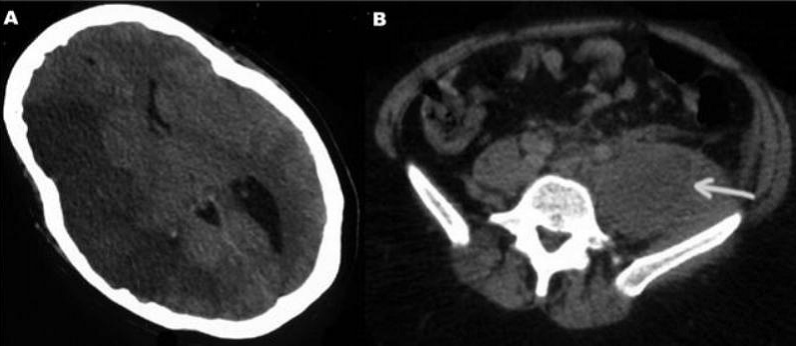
A) Brain computed tomography scan depicting a large right-sided ischemic region and cerebral edema. B) Abdominal computed tomography scans revealing multiple enlarged lymph nodes and a retroperitoneal mass adjacent to the left psoas muscle.
